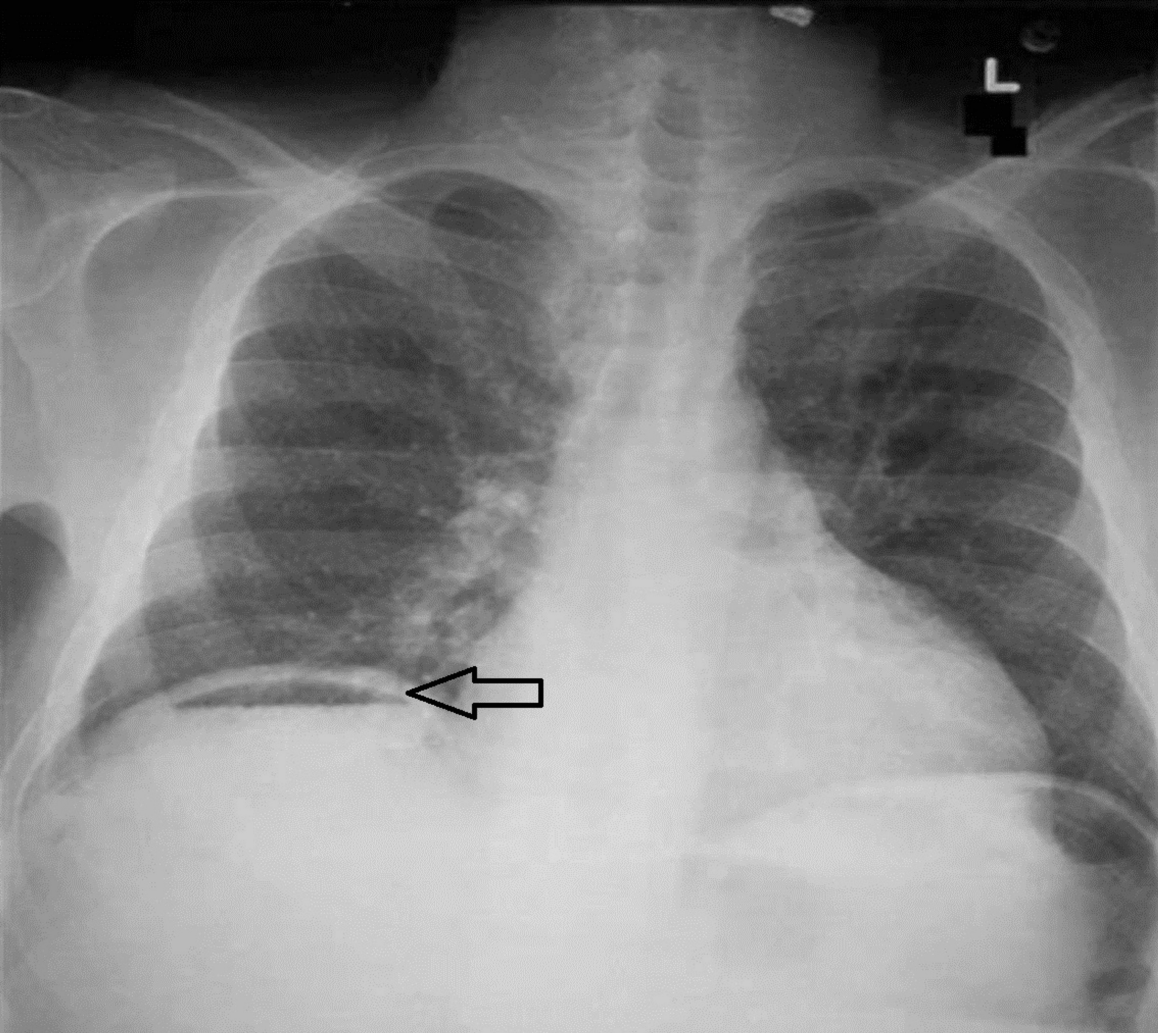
Abdominal X-ray of patient 3 showing gas under the diaphragm (arrow)
